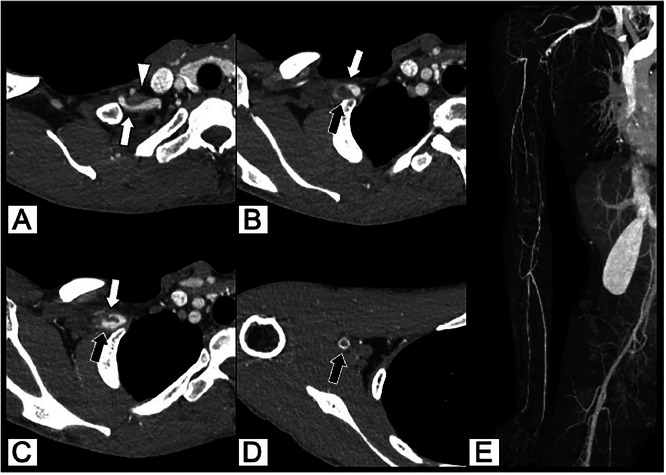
Contrast-enhanced computed tomography. (A) A stenosis is observed in the right subclavian artery (white arrow) that appears to be caused by compression of the anterior scalene muscle (arrowhead). (B-D) Post-stenotic dilatation is observed (white arrow), and a contrast filling defect suggestive of a thrombus is observed in the lumen (black arrow). (E) In the maximum-intensity projection three-dimensional reconstruction image, vascular occlusion is observed from the distal part of the right axillary artery to the brachial artery. Collateral circulation is well developed in the surrounding area, and blood flow to the forearm is maintained via collateral circulation.
